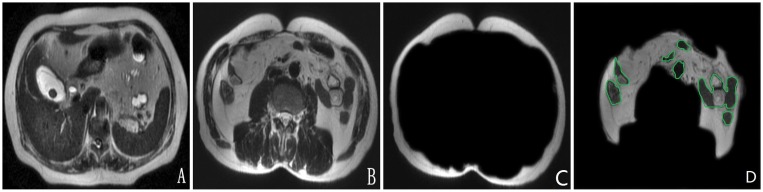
A 52-year-old male with gallbladder stone. One stone can be found in neck of gallbladder (A) and navel level image was found by a localizer sequence (B). SAT is to be the area between outline of abdominal skin and the outer abdominal muscle (C). VAT is to be enterocoelia and retroperitoneal region between the inside edge of abdominal muscles and the spinal front. Intestines and big vessels that have been circled by green line are not included in the intra-abdominal fat area (D). Axial fast-recovery fast spin-echo T2-weighted images without fat suppression shows intra-abdominal fat with SAT = 96.21 cm^2^ and VAT = 104.05 cm^2^.
